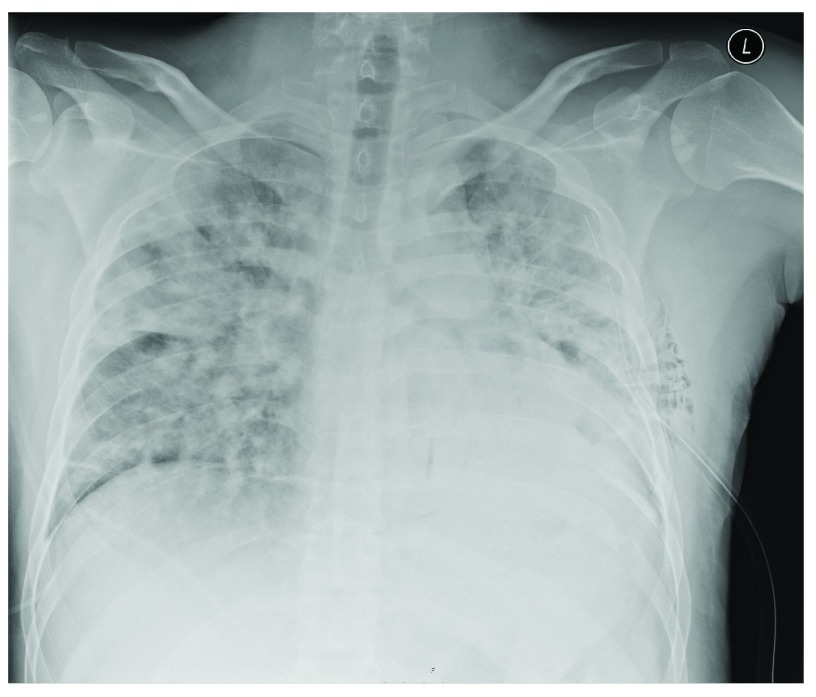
Chest radiograph one hour after drainage of the left hemopneumothorax showing bilateral pulmonary edema.
